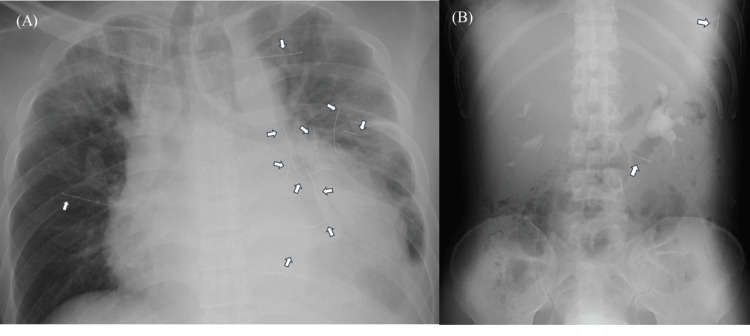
Chest and abdominal radiographs at admission. (A), (B) The chest and abdominal radiographies upon admission revealed cardiomegaly and several foreign bodies (white arrows),  consistent with retained needles in the patient’s chest and abdomen.
